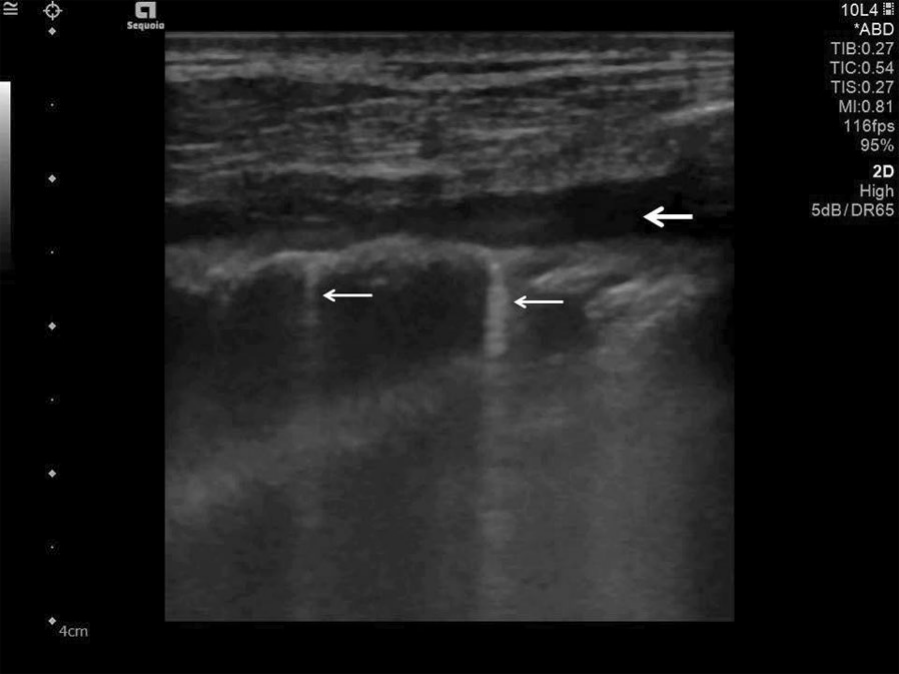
Lung ultrasonography using a linear probe. B-lines (white arrows) with irregular pleura and a small pleural effusion (bold white arrow)
